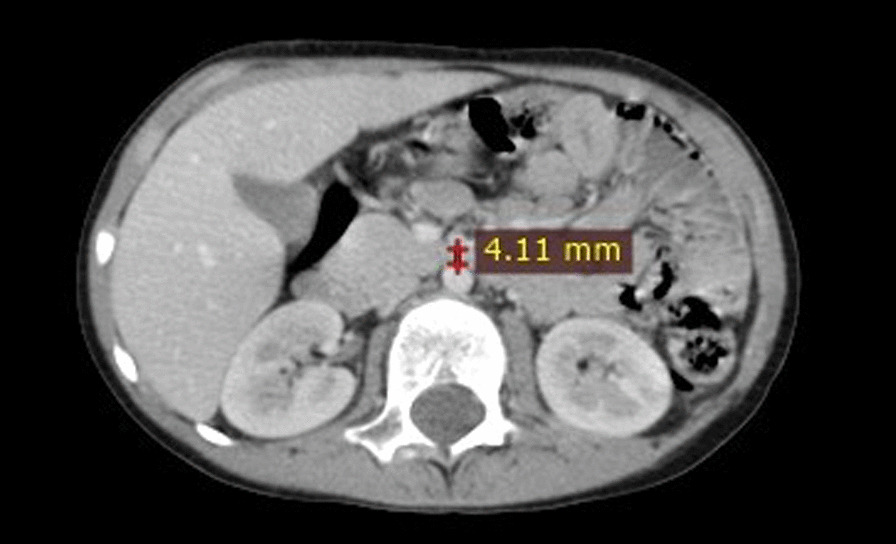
Contrast enhanced CT scan abdomen and pelvis (transverse view) showing the distance between the abdominal aorta and the superior mesenteric artery
